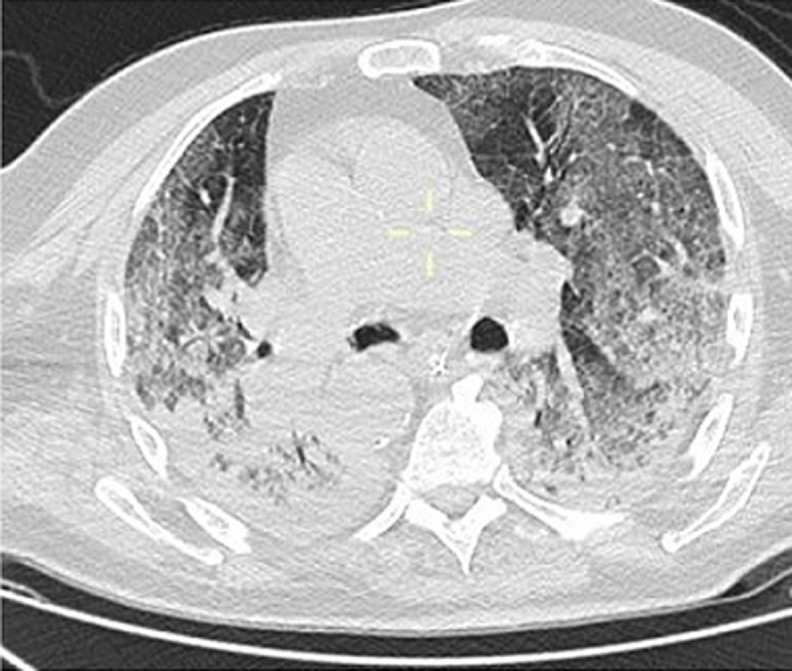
chest CT scan showing suggestive radiological finding related to COVID-19-pneumonia at the lung parenchyma including peripheral distribution of ground-glass opacities associated with consolidation
